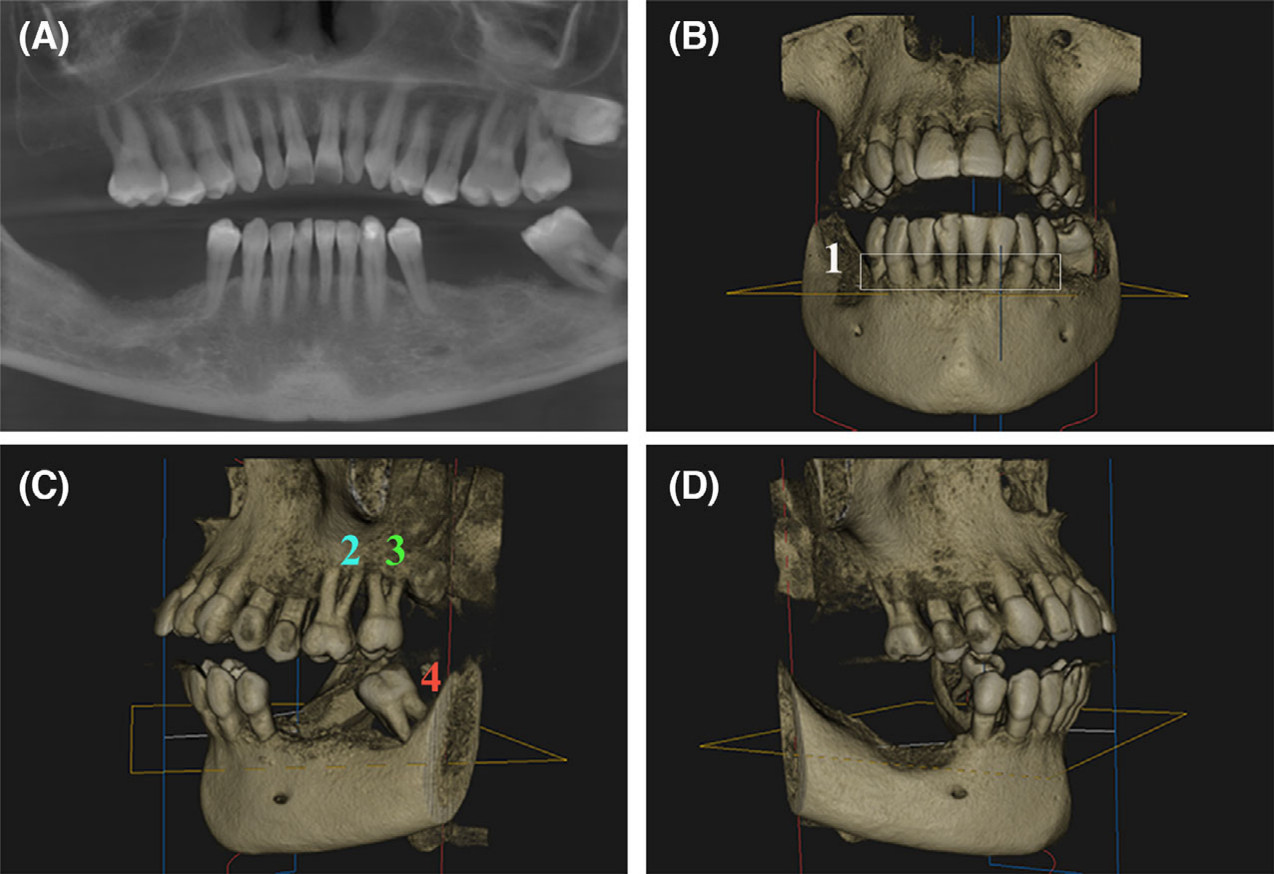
CBCT of CRC patient 89 (P89). (A) Panoramic x‐ray reconstruction obtained from the CBCT. (B) Frontal view 3D image from the CBCT. Number 1 shows a generalized horizontal bone loss affecting the lower anterior teeth with a 50% of bone loss. (C) Left lateral view 3D image from the CBCT image. Numbers 2, 3, 4 indicate areas with high grade furcation involvement. (D) Right lateral view 3D image from the CBCT image.
